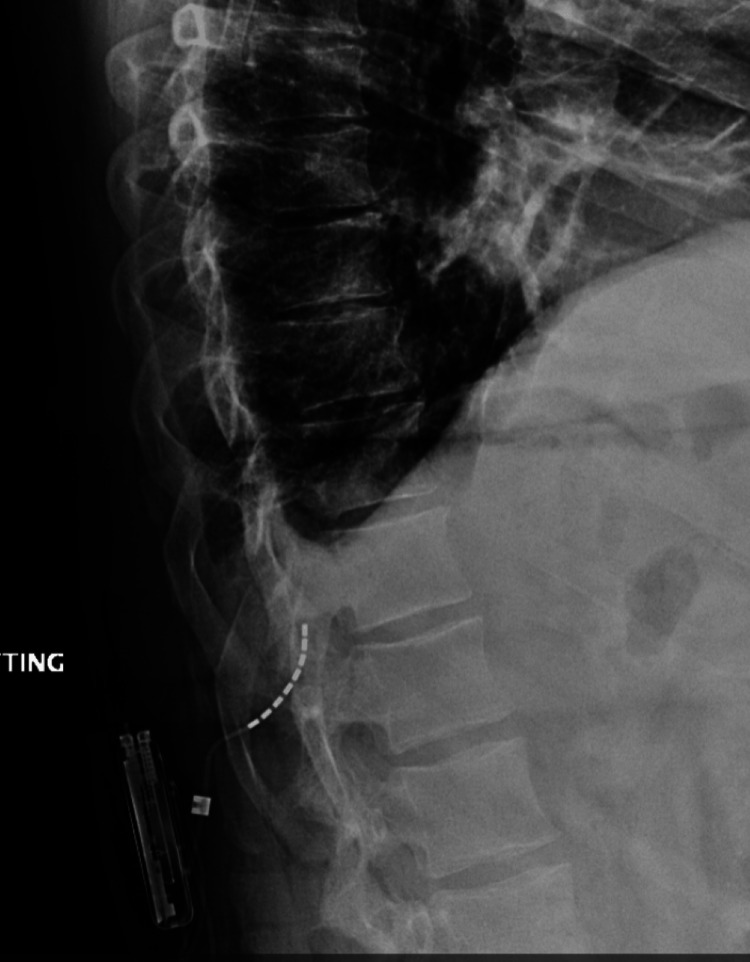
Thoracolumbar X-ray (lateral) showing lead migration
